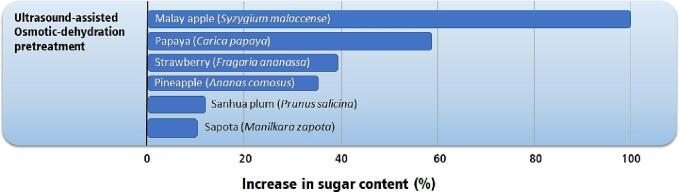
Highest sugar gain in fruits by applying ultrasound-assisted osmotic dehydration pretreatment [83], [84], [86], [91], [95], [99], [116].
